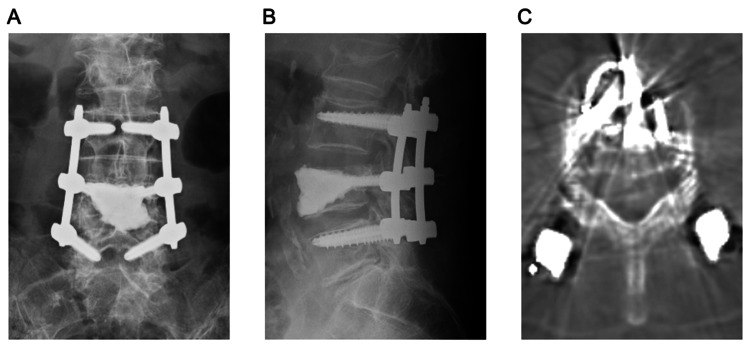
CCS placement CCS were inserted into the L4 vertebra and connected with pedicle screws positioned one level above and one level below. (A) Anteroposterior radiograph, (B) lateral radiograph, and (C) computed tomography axial view of the CCS. CCS, cement-catching screws.
